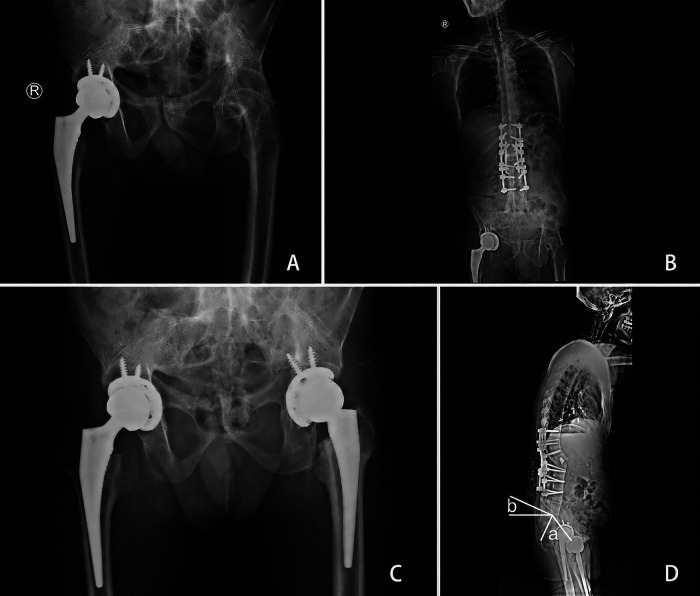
Postoperative x-rays: (A) first surgery, right THA, (B) significant improvement in scoliosis, (C) after the second THA procedure, both hip prostheses are in good position, (D) kyphosis has been effectively improved, PI a = 55°, SS b = 21°.
